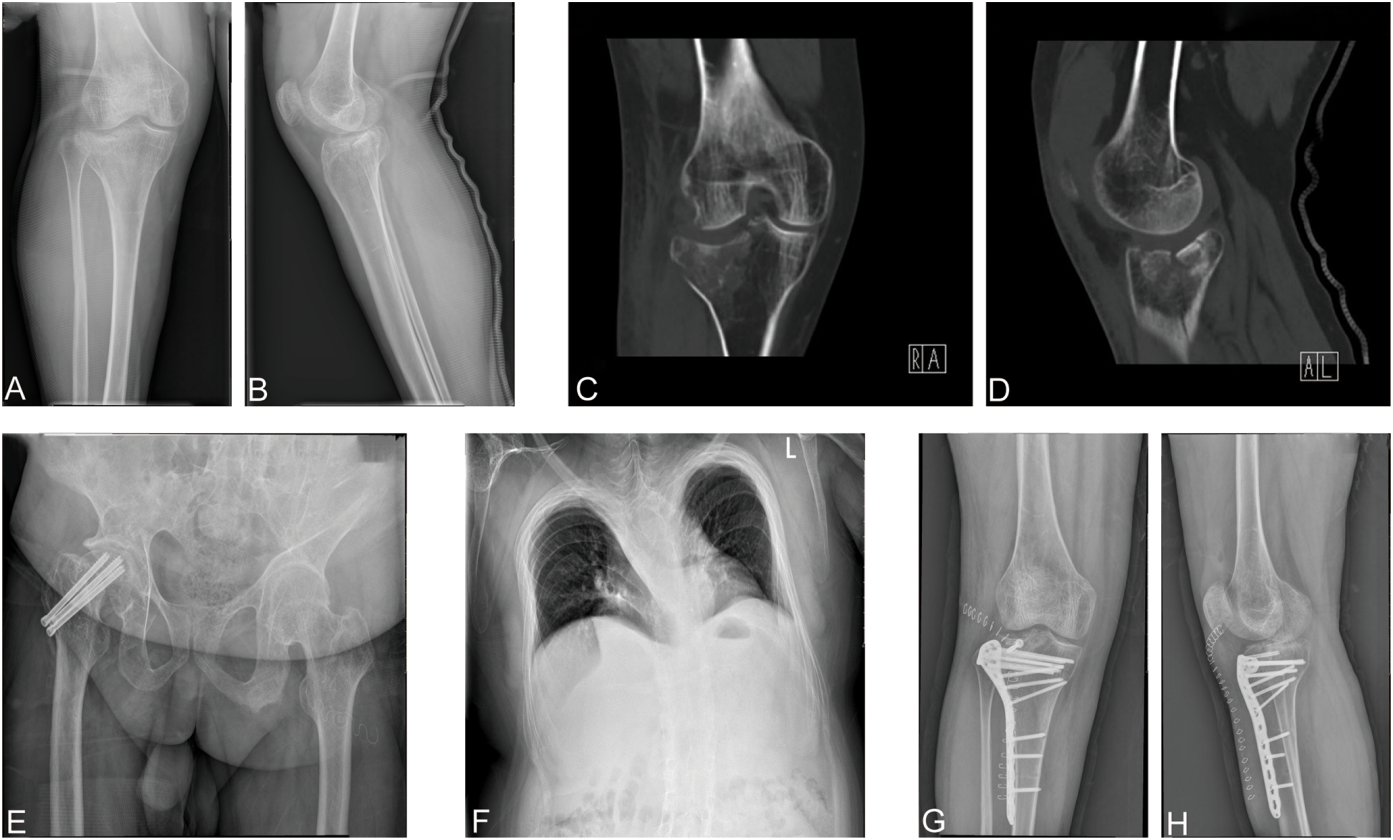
(A, B) Preoperative positive and lateral x-rays of the knee joint. (C, D) Preoperative CT plain scan of the right knee joint. (E) Anteroposition radiographs of the right hip. (F) X-ray of the chest. (G, H) Postoperative right knee joint anteroposterior and lateral x-ray showed good alignment of the broken end and internal fixation in place.
